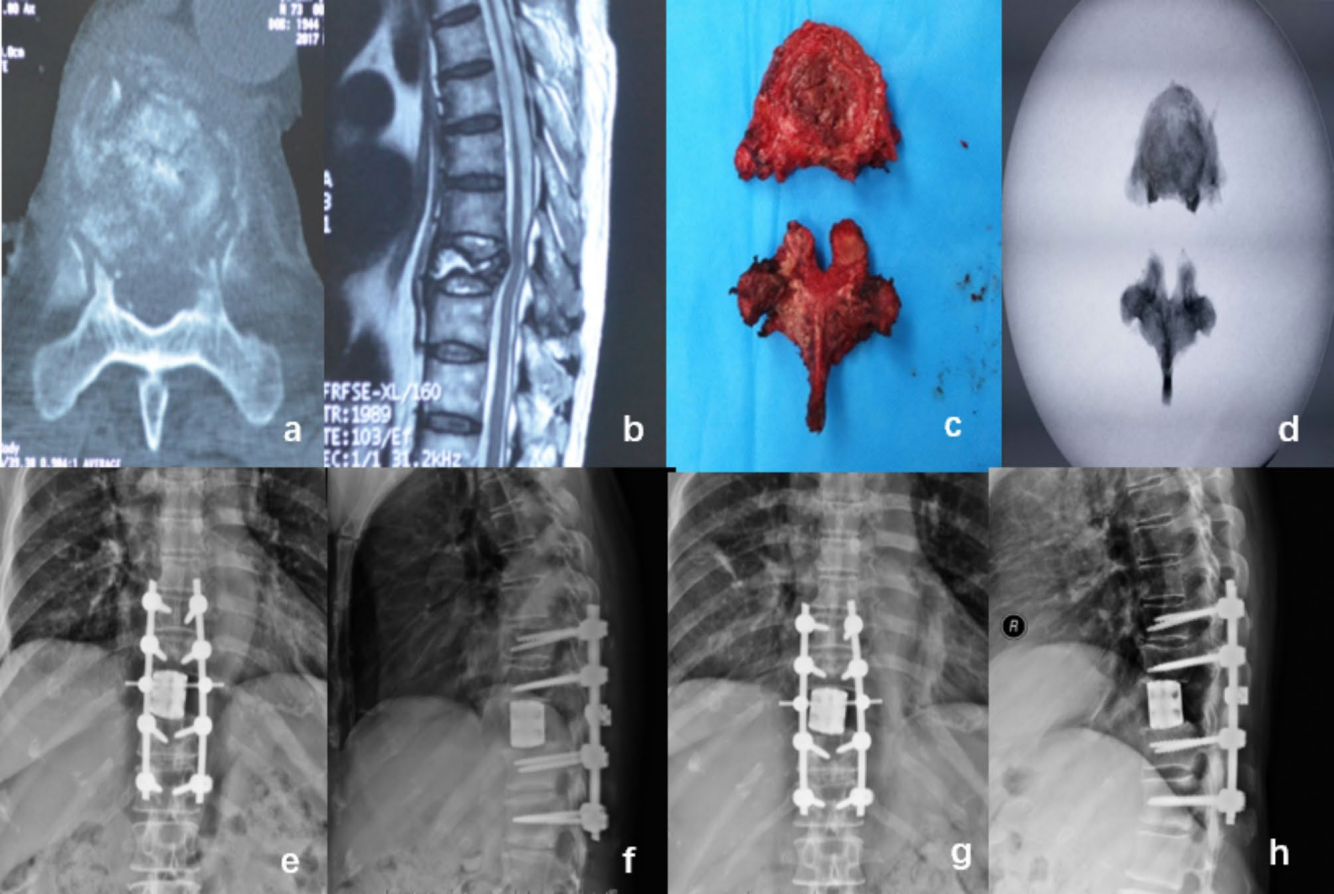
a 66-year-old female patient presented with breast cancer that had metastasized to the T9 vertebra. Preoperative CT and MRI scans disclosed a local posterior convex deformity and osteolytic destruction (a.b). The affected vertebra was completely excised during surgery(c.d). postoperative X-rays demonstrated the successful implantation of a prefabricated 3D-printed prosthesis, with notable restoration observed in the sagittal sequence (e.f). Follow-up X-rays conducted 16 months postoperatively showed no subsidence of the prosthesis(g.h)
